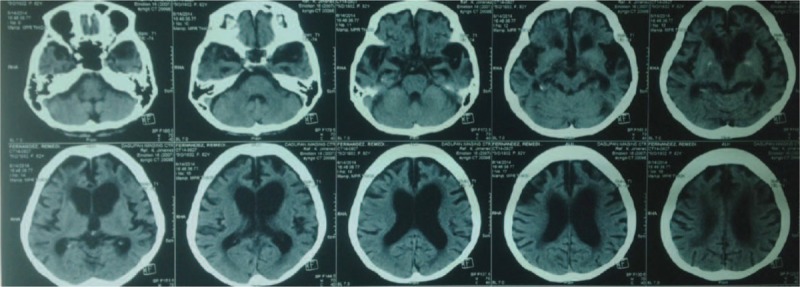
Cranial CT scan (June 15, 2014) with no evident lesions over the basal ganglia and brainstem.
